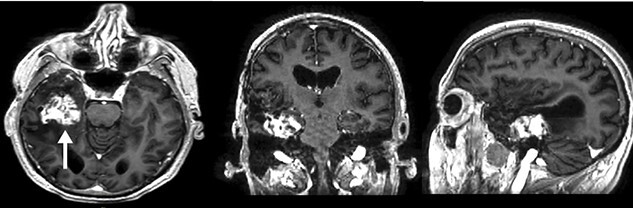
(A–C) A surveillance contrast T1W MRI (axial, coronal and sagittal) conducted at 33 months demonstrating lesional recurrence in the right temporal lobe (arrow) and minimal mass effect prior to re-operation.
